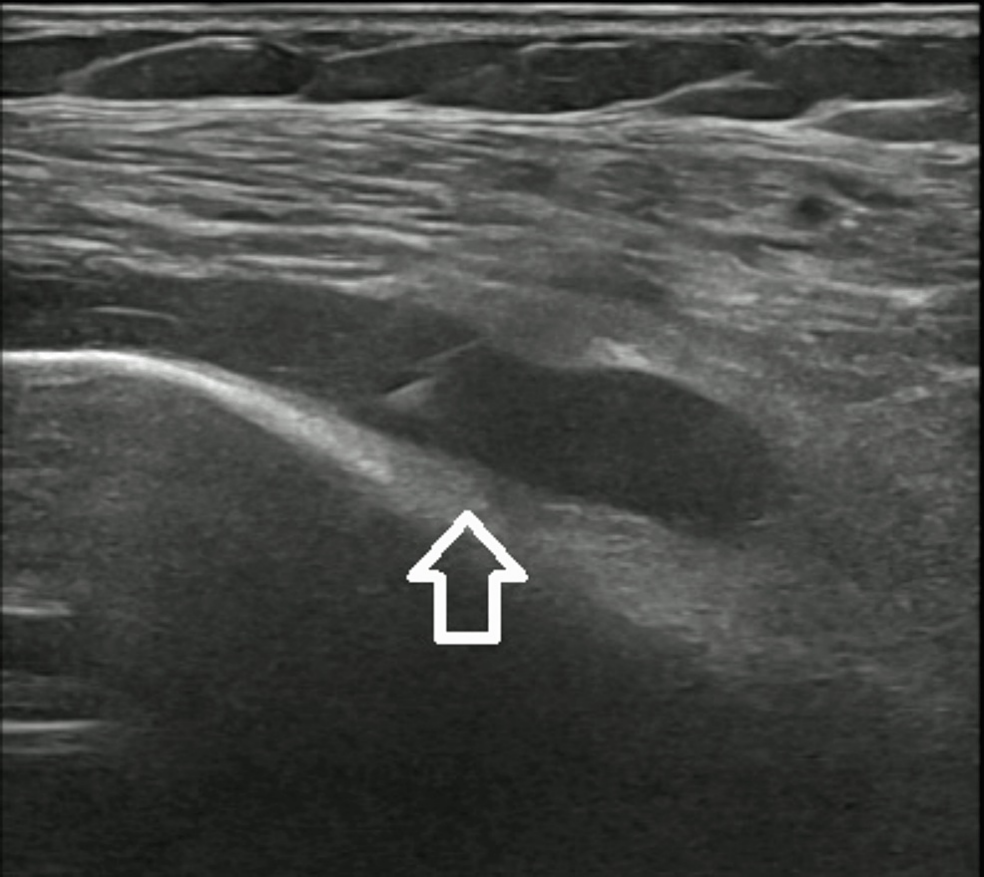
Left shoulder ultrasound revealing hypoechogenic fluid collections.
